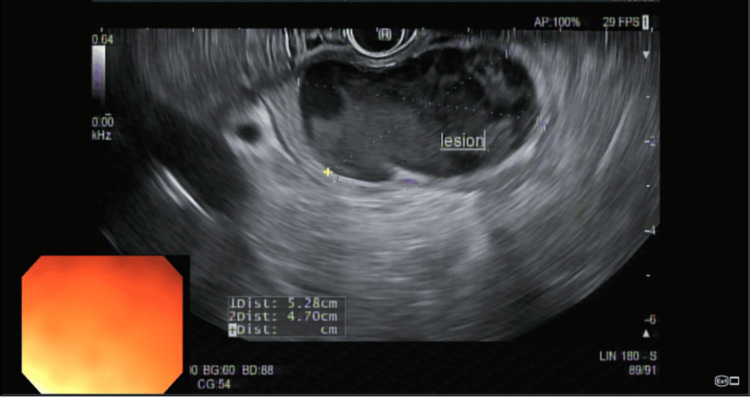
Endoscopic ultrasound view of the cystic mass. Hypoechoic and anechoic areas within a  5 × 5 cm mass seen upon ultrasound of the antrum that was later drained.
